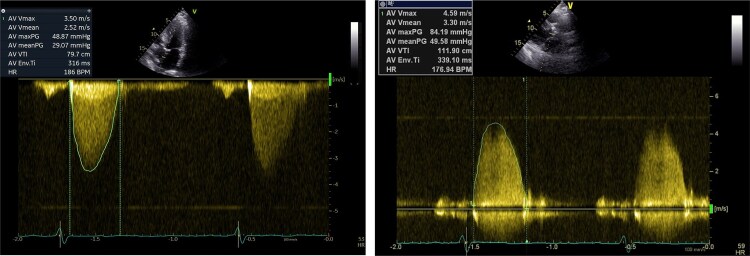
From apparent moderate towards severe aortic stenosis. In the AP5CH view, CWD interrogation yields a Pmean of 29 mmHg with an AS–VTI of 79.9 cm and an AVA of 1.2 cm^2^ suggesting moderate AS (left panel); in the modified parasternal long axis view with conventional 2D probe (right panel), a Pmean of 49 mmHg is obtained with an AS–VTI of 111.9 cm, yielding an AVA of 0.79 cm^2^, consistent with high-gradient severe AS.
